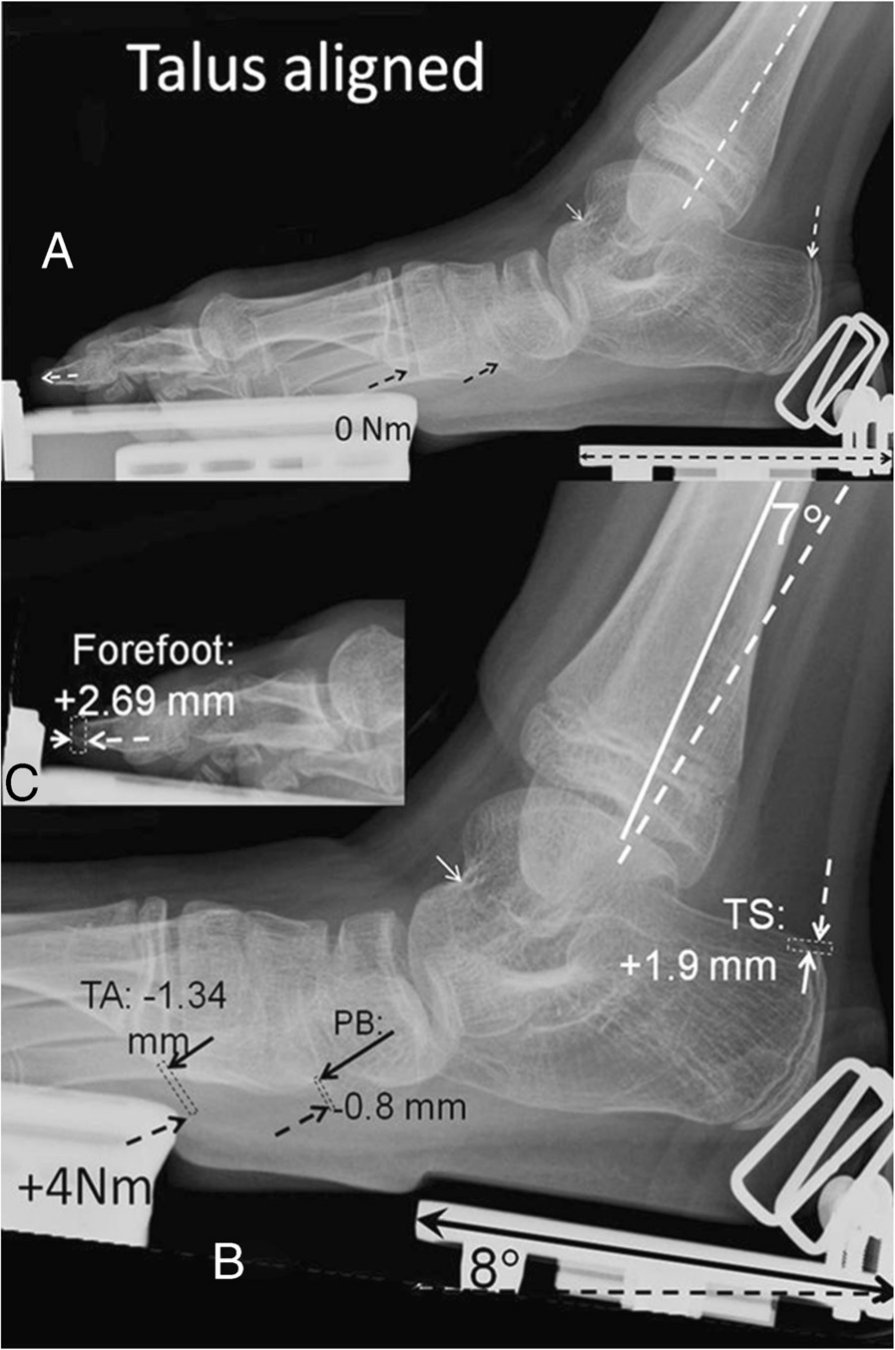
Analysis of X-rays for a SCP subject after talus alignment. A. The foot in a position similar to that when exerting 0 Nm. Under high magnification markers (dashed lines and arrows) were placed for alignment and at particular insertion points of muscles and along the fibula. Note that, for example, at the navicular bone, subluxation and an extreme position (gapping) of the calcaneo-navicular-joint is noticeable. B. The foot in a position similar to that when exerting 4 Nm dorsal flexion. The talar bone was aligned with that in A. Such talar bone alignment allows distinction of contributions to foot plate movement and muscular length changes by foot deformation and crural bone movements, respectively. The markers of A. were copied to image B. (dashed lines or arrows). New markers (solid lines or arrows) placed on the reference points in the new condition. Length changes were estimated (rectangles) for GM (m. triceps surae), TA (m. tibialis anterior) and PB (m. peroneus brevis) and angular changes for the fibula and foot plate. Note that, for clarity, magnification of A. and B. are not identical. The arrow on the heel part of the foot plate is used for calibration (actual dimension = 8.98 cm). C. Inset of the forefoot to show the presence foot lengthening upon exertion of +4 Nm.
